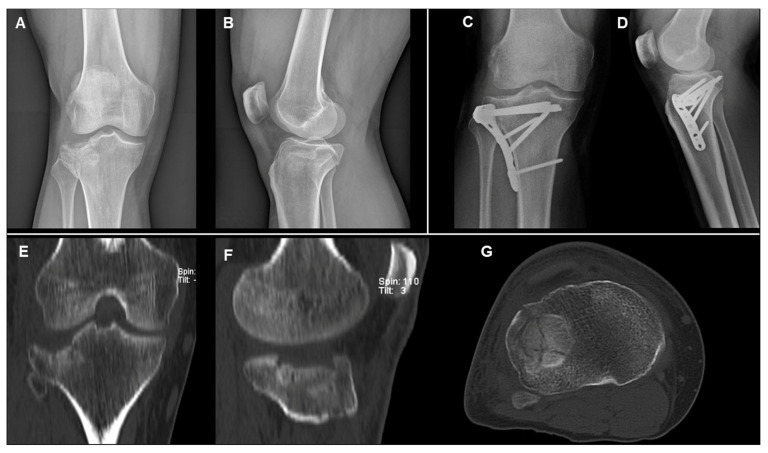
Radiographic images of a mono-condylar (Schatzker II) tibial plateau fracture in a 35-year-old female patient: AP and LL views of X-rays at preoperative period (A,B) and at 58-month follow-up (C,D), respectively. Preoperative CT scan images in coronal (E), sagittal (F), and (G) axial views, respectively.
